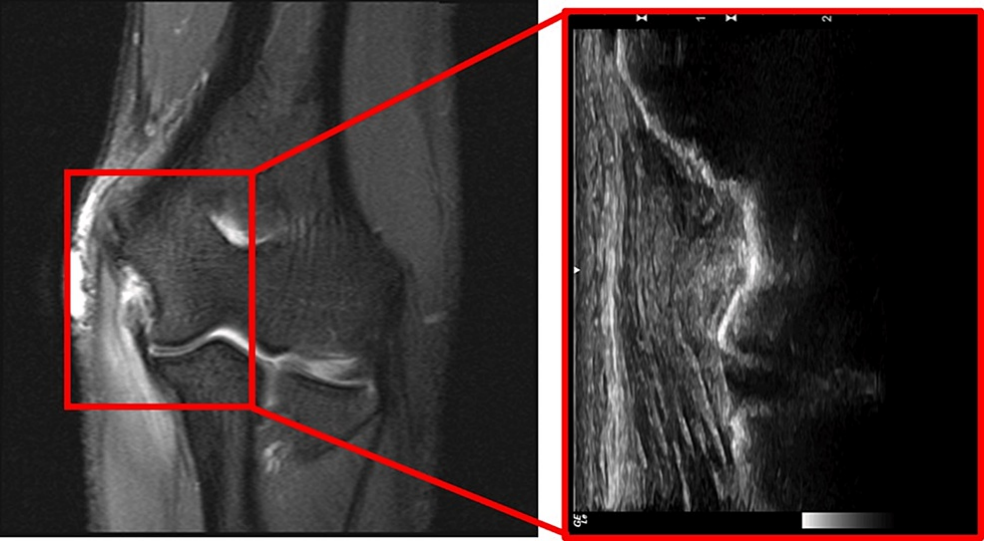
Magnetic Resonance Imaging and Ultrasound of the right elbow. Magnetic resonance imaging (MRI) demonstrates a near full-thickness, at least high-grade partial, tearing of the anterior bundle proximal portion of the ulnar collateral ligament (UCL) attachment on the distal aspect of the medial epicondyle. The zoomed image is from the associated diagnostic ultrasound which demonstrates hypoechoic thickening and loss of normal ligamentous echotexture involving the proximal portion of the anterior band of the ulnar collateral ligament.
